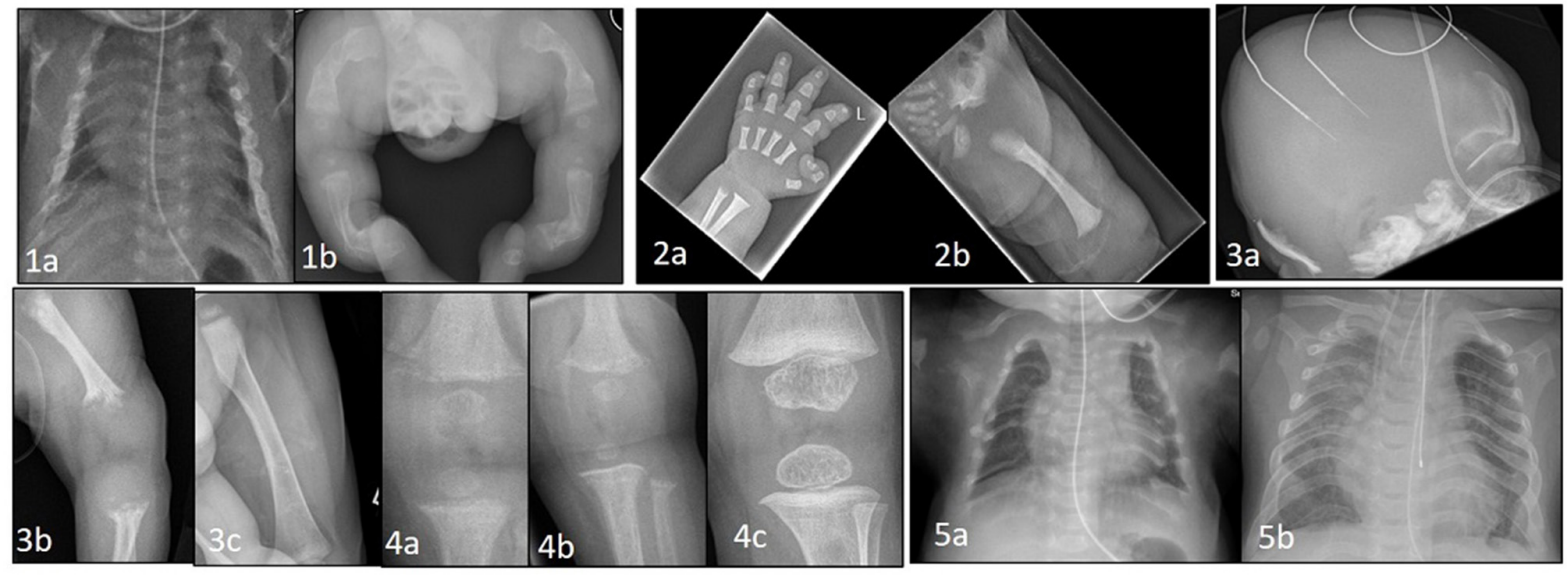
(1a) Thin, gracile and beaded ribs in a case of severe osteogenesis imperfecta at birth. (1b) Lower limbs showing bowing and deformity of long bones with multiple fractures in various stages of healing. (2a) Hand x-ray in achondroplasia showing short metacarpals and phalanges with trident sign. (2b) Hip shows classic features of horizontal acetabular roof, squared off iliac crest, small sacrosciatic notch, and scalloping of proximal femur. (3a) Perinatal hypophosphatasia at birth showing severe skull hypomineralization. (3b) severe hypomineralization of long bones and metaphyseal lucencies. (3c) Healing of metaphyseal lesions and markedly improved mineralization after 12 months of asfotase alfa treatment. (4a) Knee x-ray in a case of NHSPT at 3 months showing metaphyseal irregularity, coarsening of trabeculae, and subperiosteal resorption. (4b) progressive changes at 6 months, and (4c) complete healing following total parathyroidectomy. (5a) Chest X-ray at birth in a baby with TRPV6 mutation. Ribs are malformed with severe demineralization. (5b) Spontaneous improvement at 2 years of age with remodeling, improved mineralization of ribs and thoracic volume.
